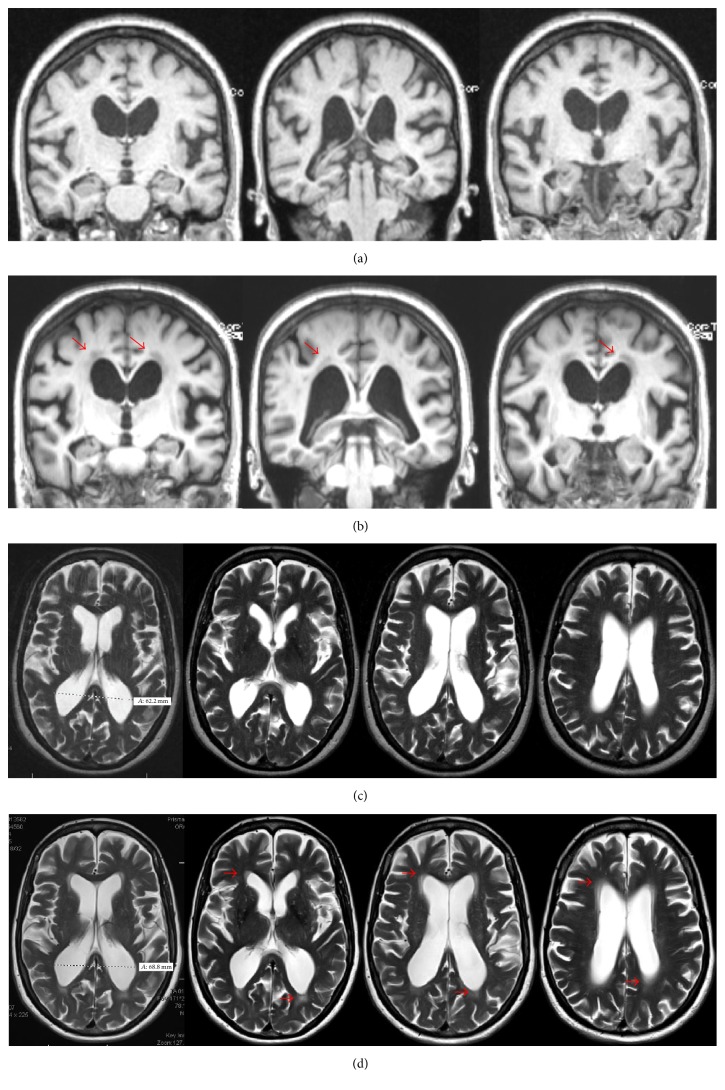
Brain MRI imaging. (a) Baseline coronal MPR sequences. (b) One-year follow-up coronal MPR sequences: periventricular white matter hypointensity (red arrows). Slight narrowing of cortical gyri on the vertex with enlarged Sylvian fissure. (c) Baseline Axial T2-weighted sequences. (d) One-year follow-up axial T2-weighted sequences: increased periventricular white matter hyperintensity (red arrows).
